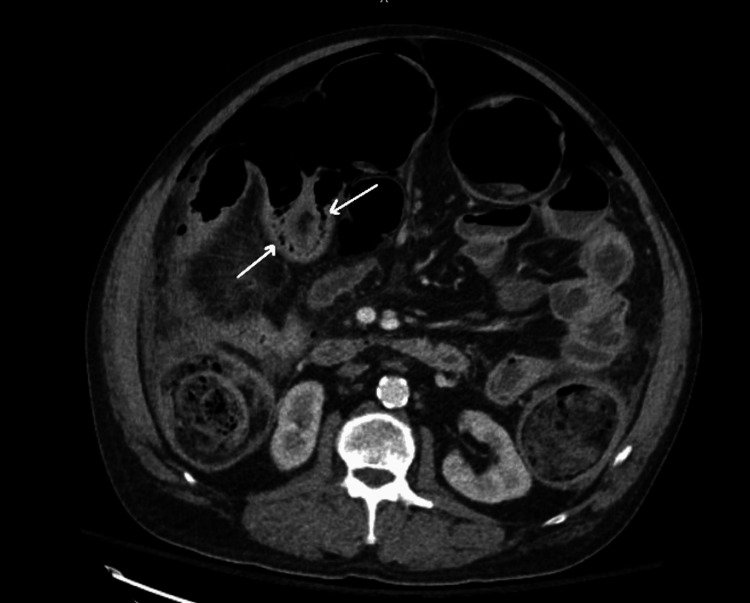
CT abdomen and pelvis with contrast - image 2 White arrows indicate air within the wall of the large bowel (pneumatosis). There is also severe dilation of the large bowel with air fluid levels consistent with an obstruction CT: computed tomography
